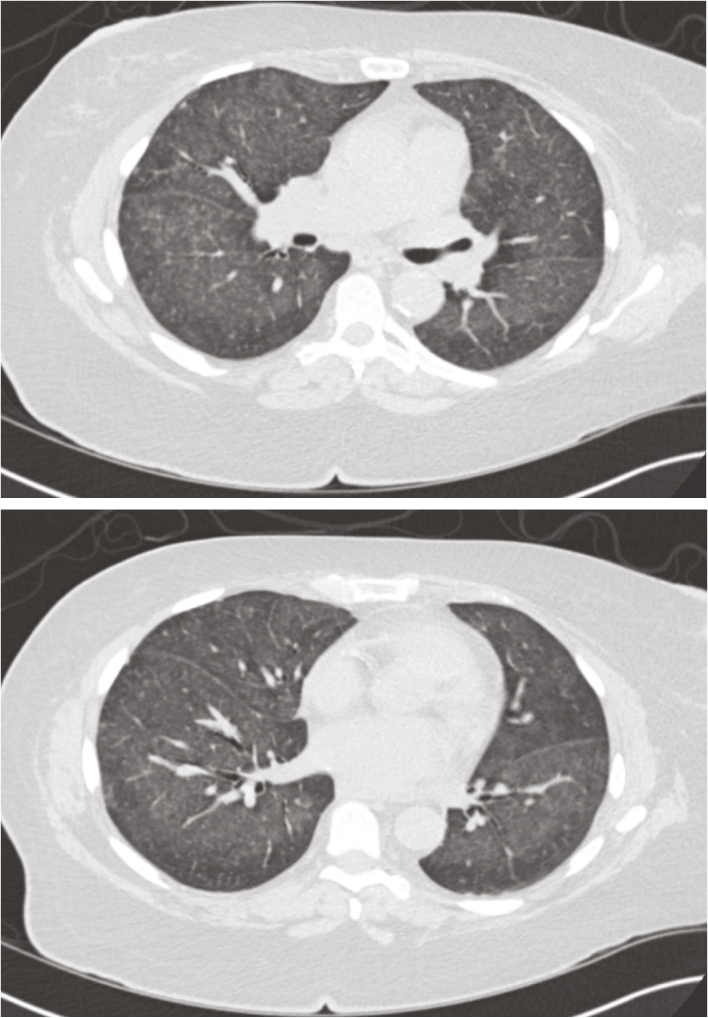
Admission noncontrast chest CT demonstrating diffuse confluent ground-glass opacities in all five lobes with prominent subpleural sparing.
